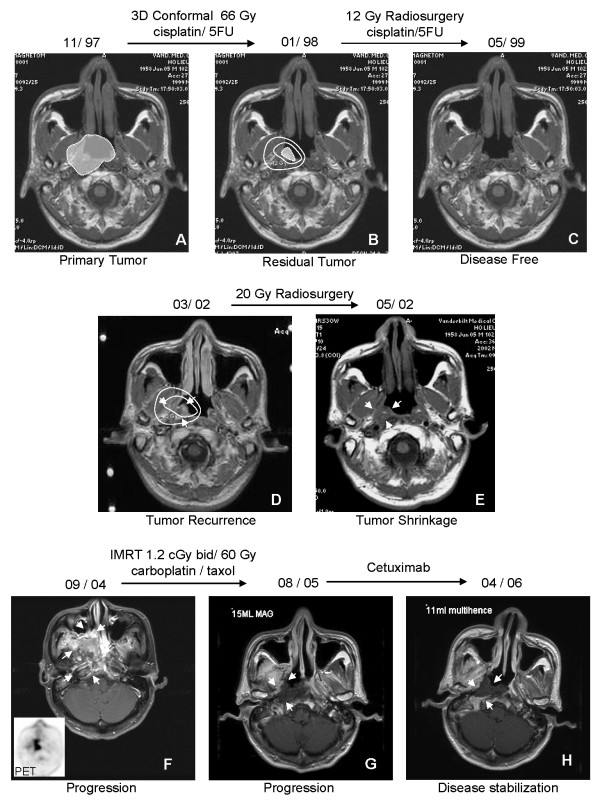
Multiple MRI scans of the nasopharynx listed in chronological order showing the progression of disease after multiple treatments. In Part A and B, the extent of the tumor was indicated with the white enclosed areas. In Part C to H, boundaries of the tumor are indicated (arrows). Dosimetries of the radiosurgery are indicated in Part B and D (concentric curved circles). Part F contained FDG-PET scan of the same period in the same nasopharyngeal plane as the MRI.
